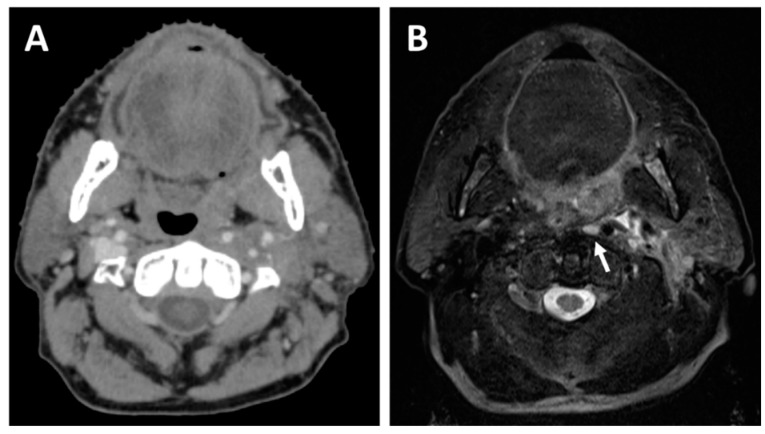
A 64-year-old patient with a cT2N1 p16-positive squamous cell carcinoma of the left tonsil planned for concurrent chemoradiotherapy. The planning CT (A) shows a mass effect originating from the left tonsil, whereas the planning T2-weighted MRI (B) allows better visualization of the left tonsil tumor and also detects a left retropharyngeal lymph node metastasis (white arrow).
